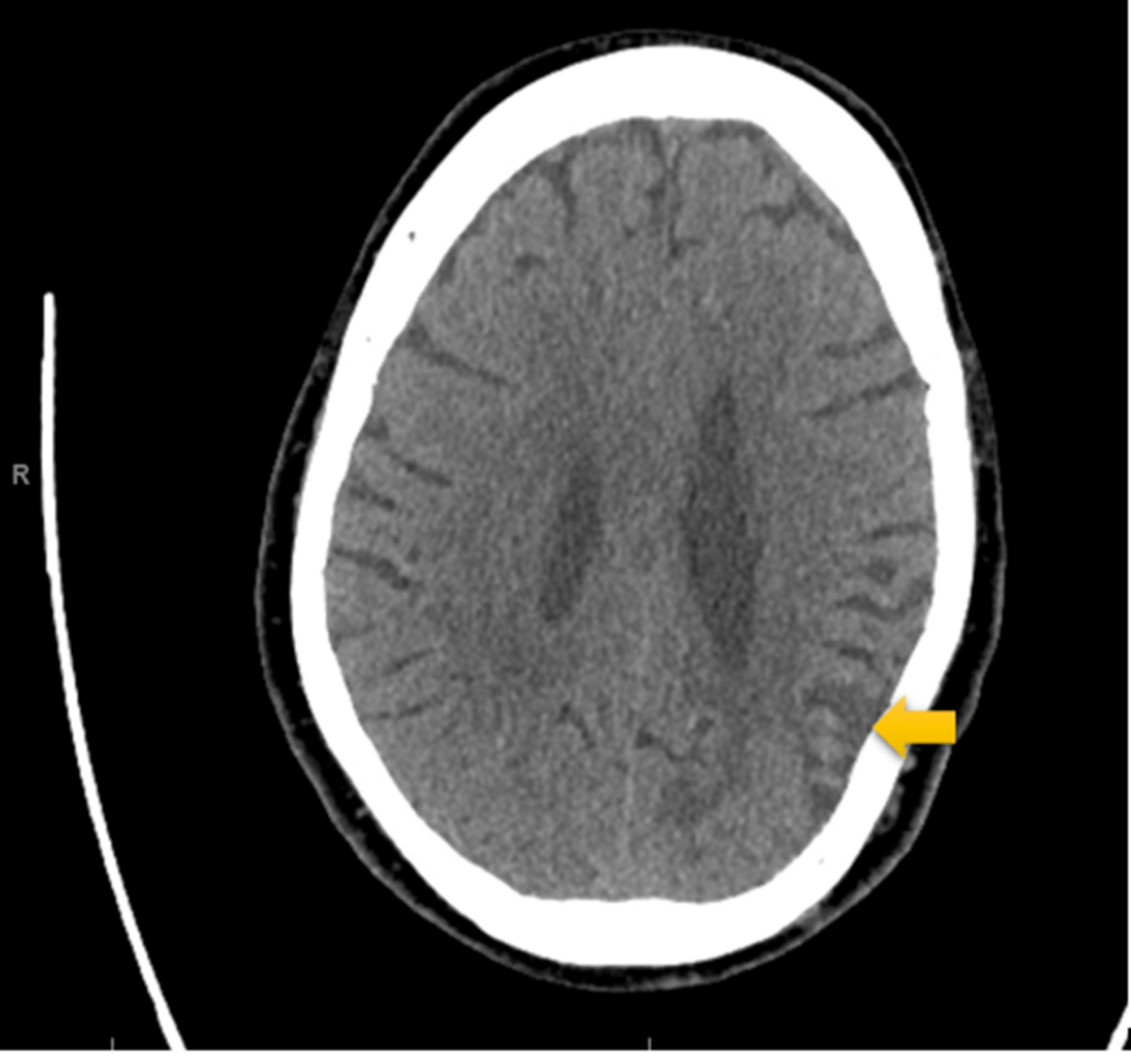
Non-contrast CT Scan of Head A non-contrast CT scan of the cerebral cortex from a few months prior to this hospitalization. Remote areas of infarction were described in the left parieto-occipital lobe.
